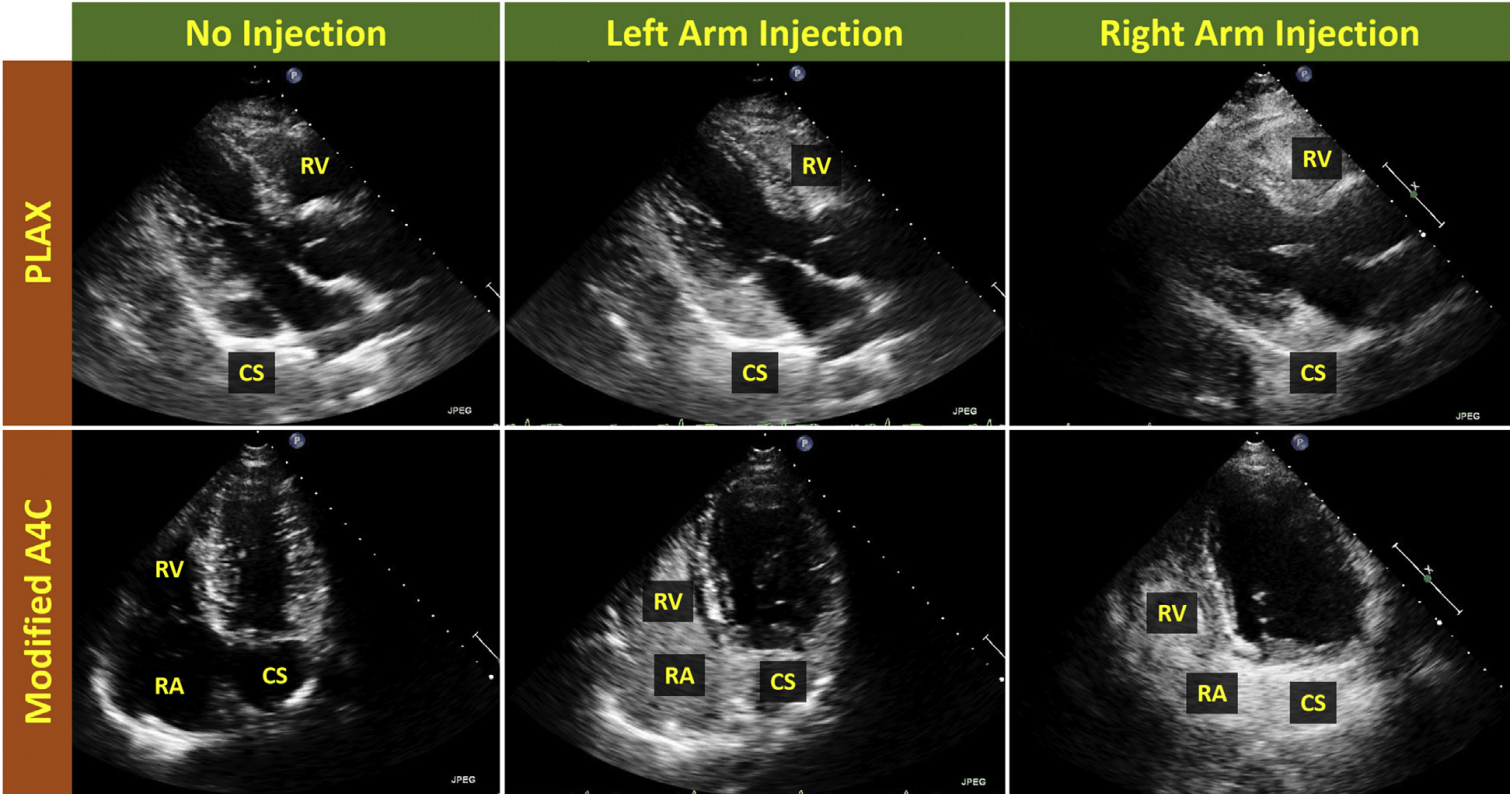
Case 2: persistent L-SVC, absent R-SVC. Transthoracic echocardiogram in PLAX and modified A4C views with intravenous agitated saline injection in left and right arms. After left arm agitated saline injection, the pattern of opacification (dilated CS first, right heart next) is consistent with the diagnosis of a persistent L-SVC and is similar to the opacification pattern seen in Figure 2. After right arm agitated saline injection, the pattern of opacification is the same as after left arm injection. This suggests the absence of an R-SVC. Videos 2 and 3 correspond to this figure. *A4C*, Apical four-chamber; *CS*, coronary sinus; *PLAX*, parasternal long axis; *RV*, right ventricle.
